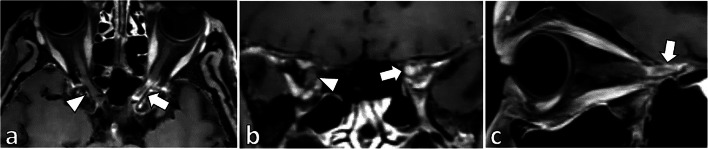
Left pre-chiasmatic optic nerve enhancement in a patient diagnosed with GCA. (a) Axial, (b) coronal, and (c) sagittal postcontrast BB-MRI images show contrast enhancement and inflammation of the left pre-chiasmatic optic nerve on BB-MRI (a–c, arrows), which contrasts with the normal right pre-chiasmatic optic nerve (a and b, arrowheads)
